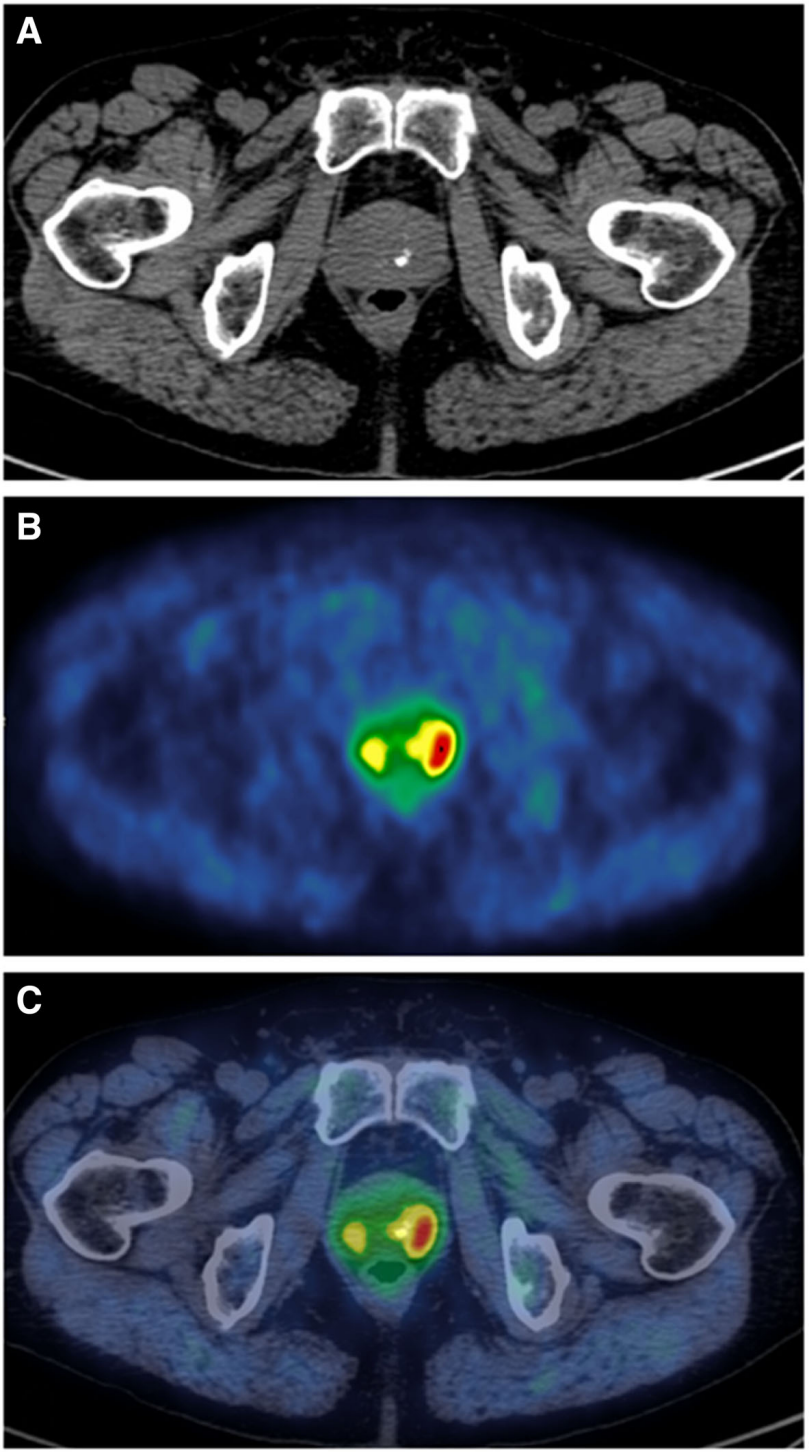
Focal uptake detected on the periphery of the left prostatic lobe. a CT scan; b 11C-choline PET scan; c 11C–choline PET/CT fused scan
